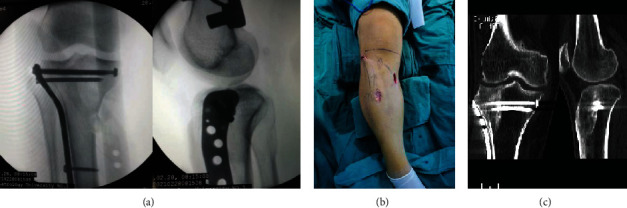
Lateral plate and screw fixation (Schatzker type II) was performed using the minimally invasive percutaneous plate osteosynthesis (MIPPO) technique. (a) Intraoperative fluoroscopy was performed after fixation; (b) surgical incisions; (c) CT scans of the knee during postoperative follow-up.
